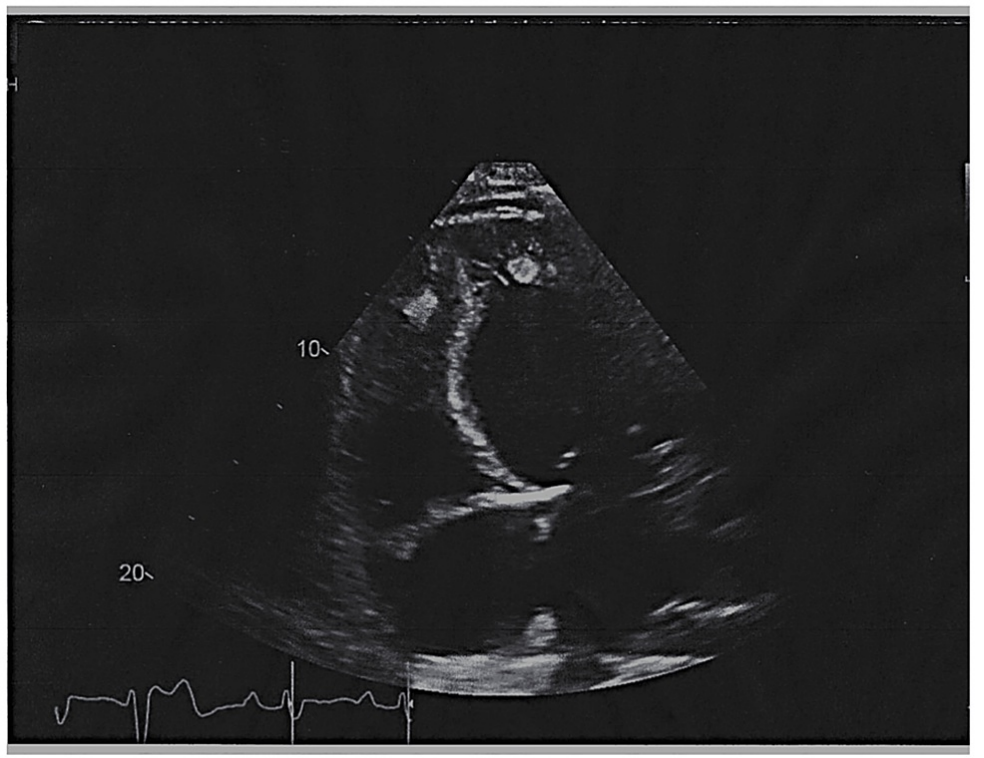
Echocardiogram showing cardiomyopathy with biventricular thrombi.
